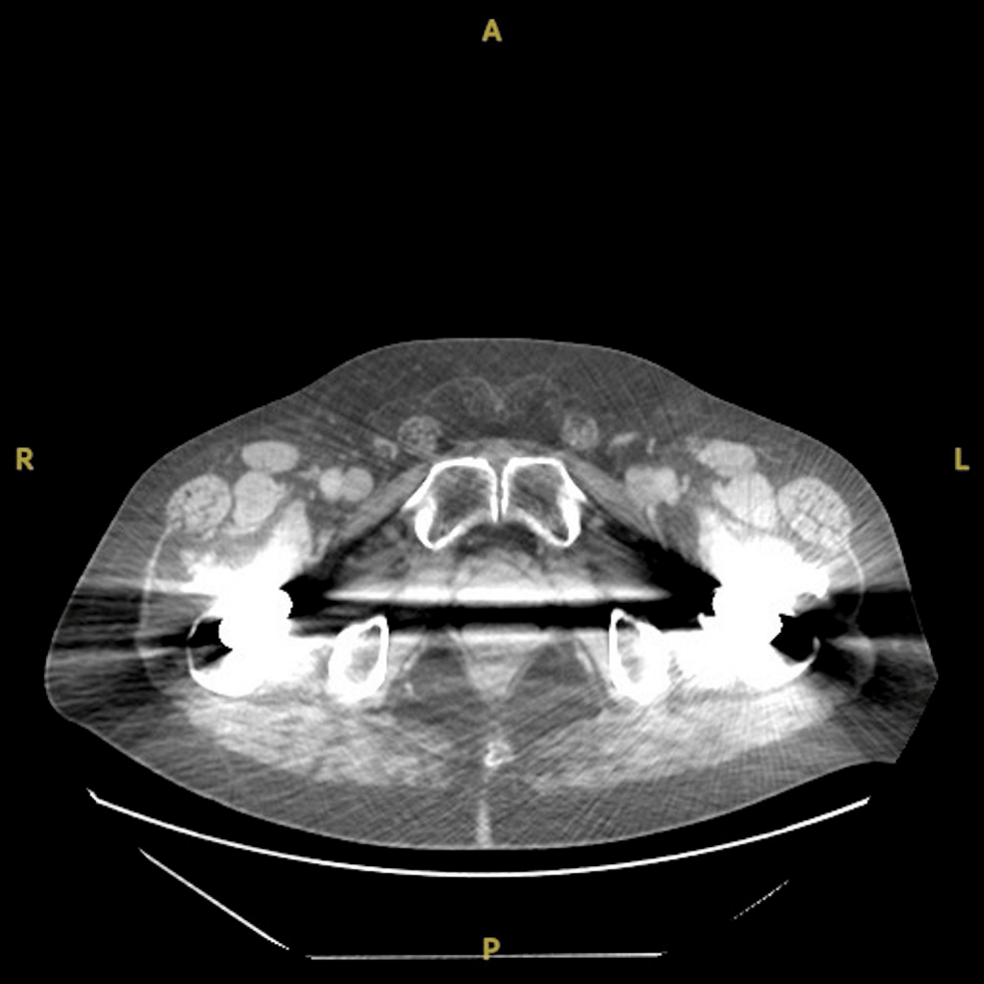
Post-salvage radiotherapy CT chest/abdomen/pelvis showing the complete eradication of inguinal disease. CT: computed tomography
